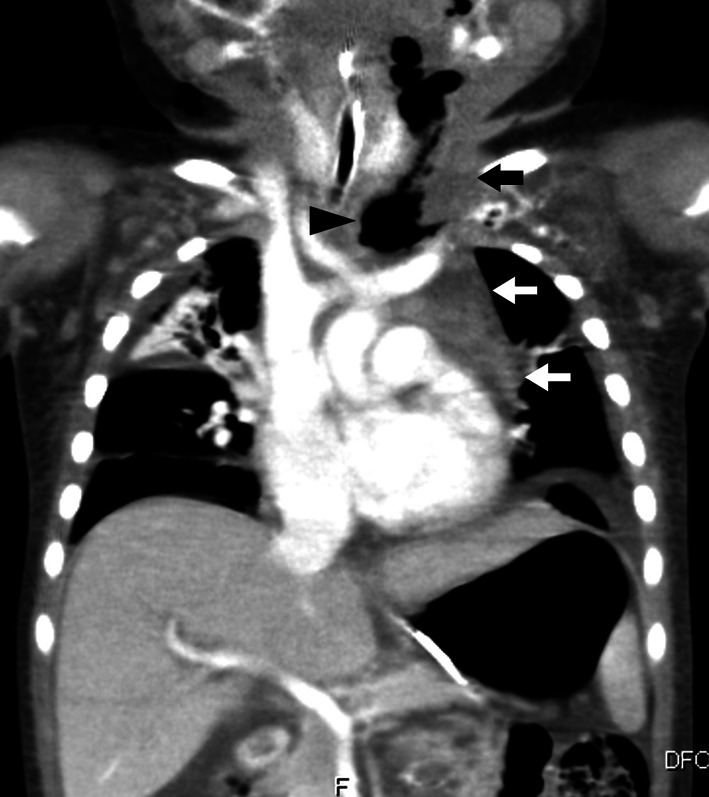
Chest CT scan on day 3 of admission. A CT scan confirmed the presence of deep abscesses (arrow) in the cervical and upper posterior mediastinum with pneumomediastinum (arrowhead)
